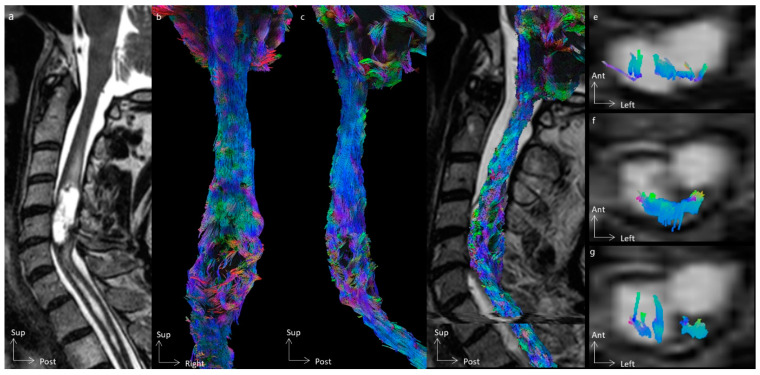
Spinal cord tractography in patient 3, with an ependymoma, grade II. (a) Sagittal T2-weighted image showing a cystic C4–C5 intramedullary tumor, whose cystic walls are enhanced after gadolinium injection. (b,c) Ventral and lateral views of the spinal cord tractography, showing white fiber disorganization at the tumor level. (d) Overlay of the spinal cord tractography on the sagittal T2-weighted MR images. (e) Overlay of the corticospinal tract on the axial T2-weighted MR images, within the tumor. (f) Overlay of the dorsal columns on the axial T2-weighted MR images, compressed dorsally, and within the tumor on the left side. (g) Overlay of the spinocerebellar tracts on the axial T2-weighted MR images.
